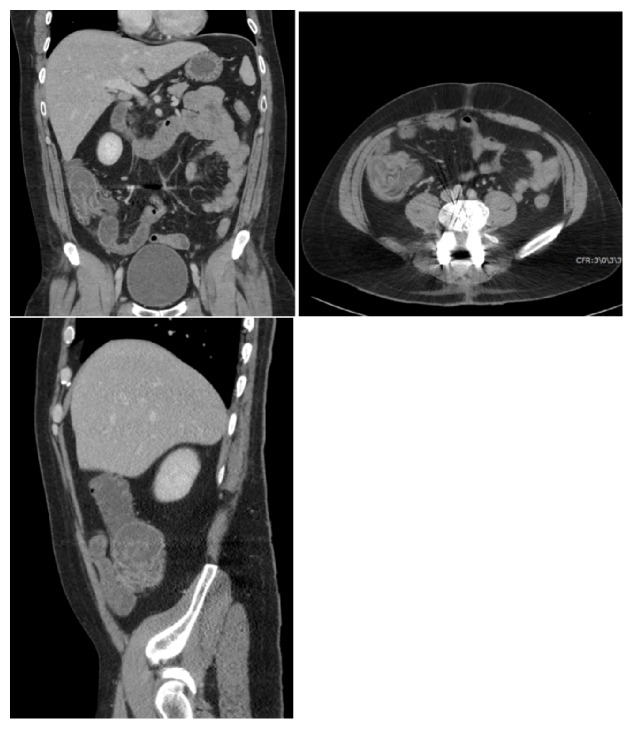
Coronal, axial, and sagittal contrast enhanced CT image demonstrates a polypoid mass in the base of the cecum representing a cecoappendiceal intussusception, mimicking a polyp or neoplasm.
